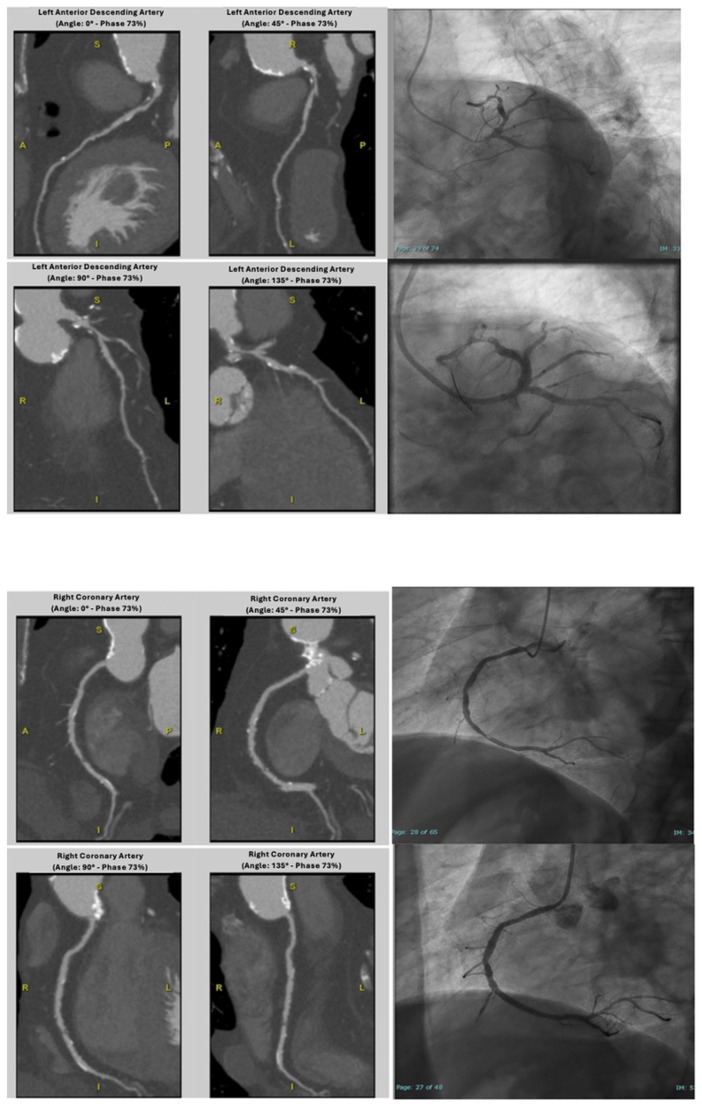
CT reconstructions of coronary arteries and angiographic findings before and after PTCA. On CTA: short common trunk with calcified plaque causing a 40–50% stenosis. At the level of the proximal LAD, fibrolipidic plaque with severe stenosis (>70%; minimal luminal area of approximately 1 mm^2^). RCA with diffuse atherosclerosis and focal fibrolipidic plaque in its distal segment causing subocclusive luminal stenosis. These alterations were confirmed by an angiographic exam and treated with PTCA. PTCA = Percutaneous Transluminal Coronary Angioplasty, LAD = Left Anterior Descending artery, RCA = Right Coronary Artery.
